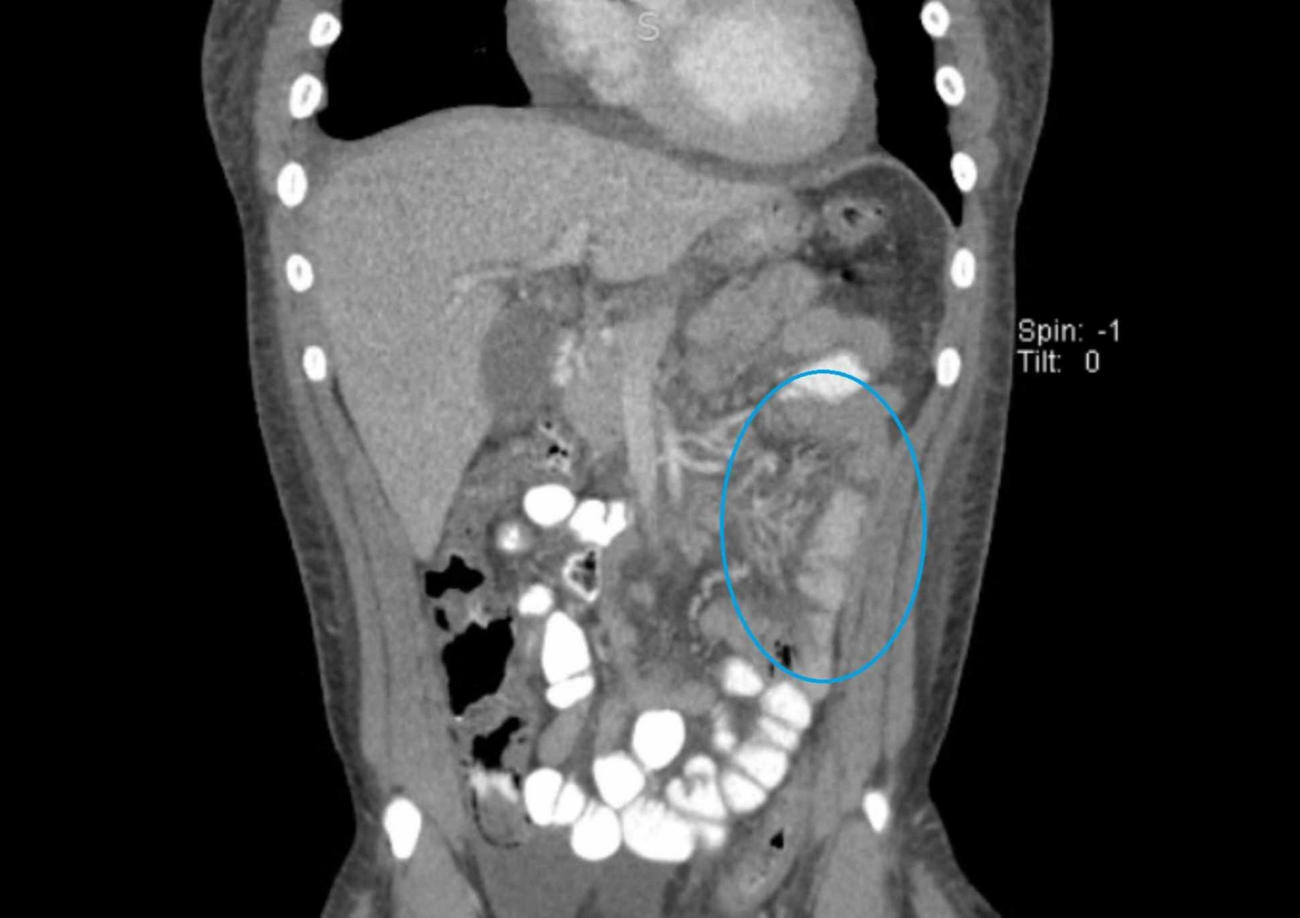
Combs sign on CT of the abdomen (blue oval) CT: computed tomography
